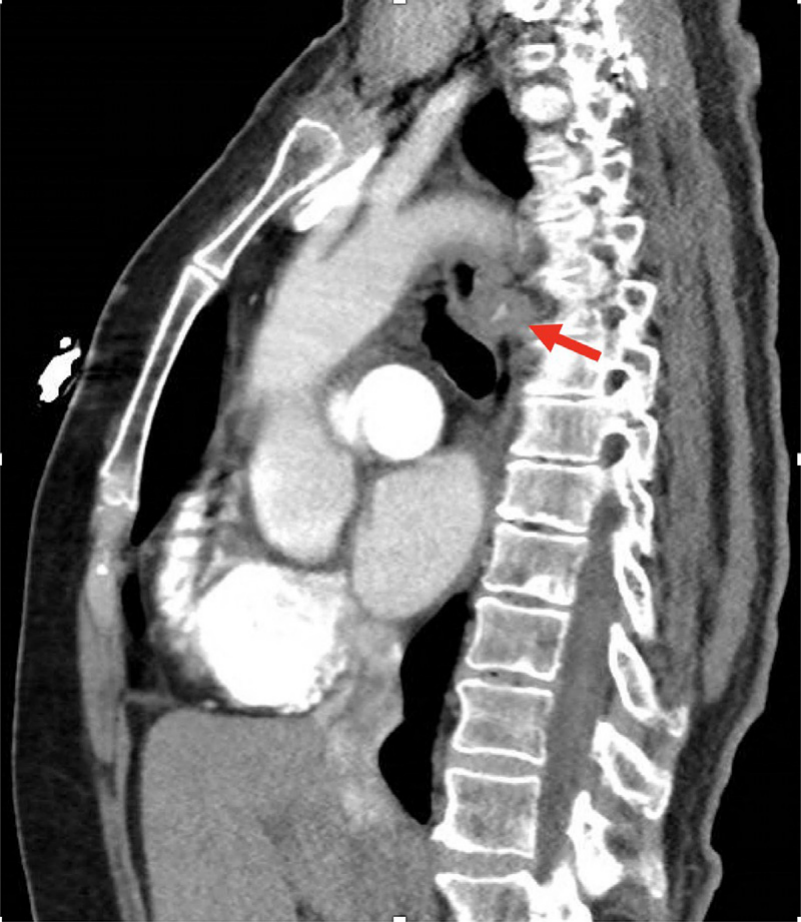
Sagittal view of CT chest with arrow pointing to a mass present in the posterior esophageal wall.
